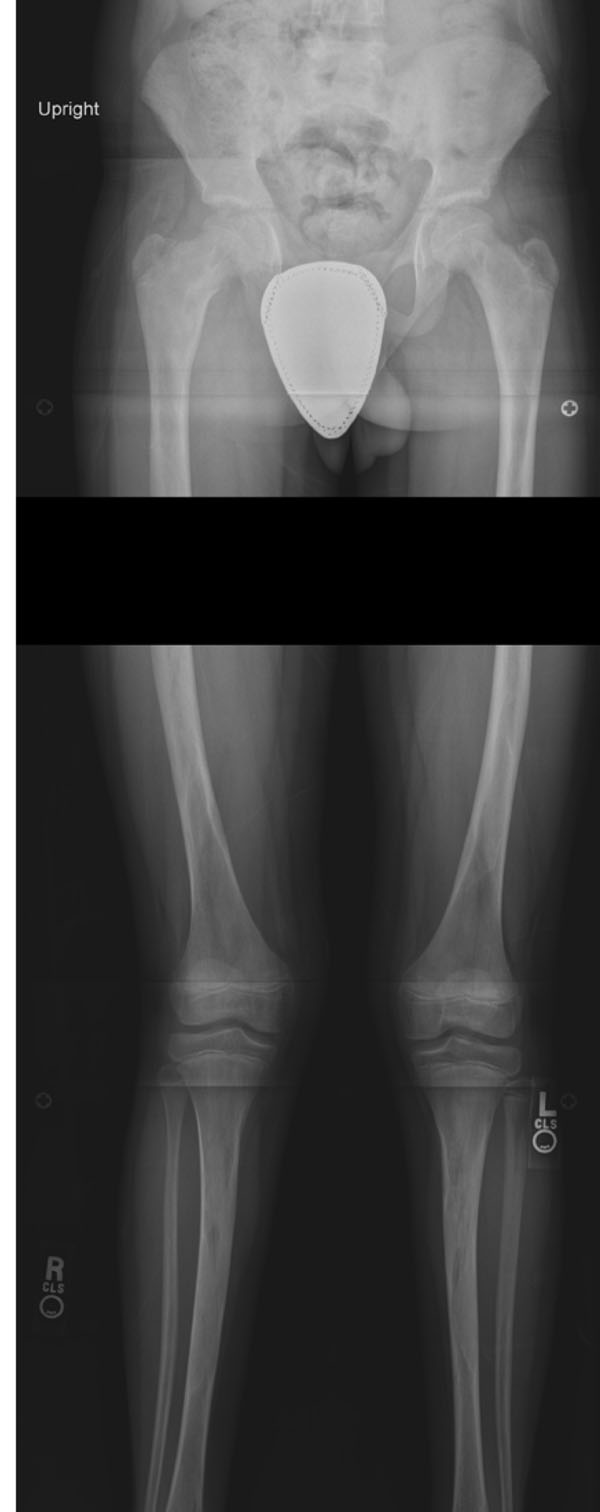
Long standing anterior/posterior (A/P) radiograph. This single view A/P film of both extremities with the patient standing allows for assessment of the extent of FD in both legs, hip angulation, and for potential limb length discrepancy. This radiograph demonstrates involvement of both femurs, both tibias, and early deformity of the upper right femur with decreasing neck-shaft angle.
